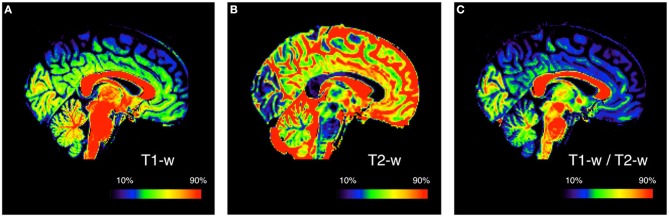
Myelin enhanced contrast image for a representative subject. The ratio of T1-w (A) to T2-w (B) signal intensity is calculated to obtain the T1-w/T2-w ratio image (C). This is done to improve the mapping by increasing the contrast between different myelinated structures. Since conventional MRI images have arbitrary intensity scales, the three images are showed with a colormap assigned on the basis of the 10 and 90th percentile values. The subject used for this figure is Subject 30 of the KIRBY21 database.
